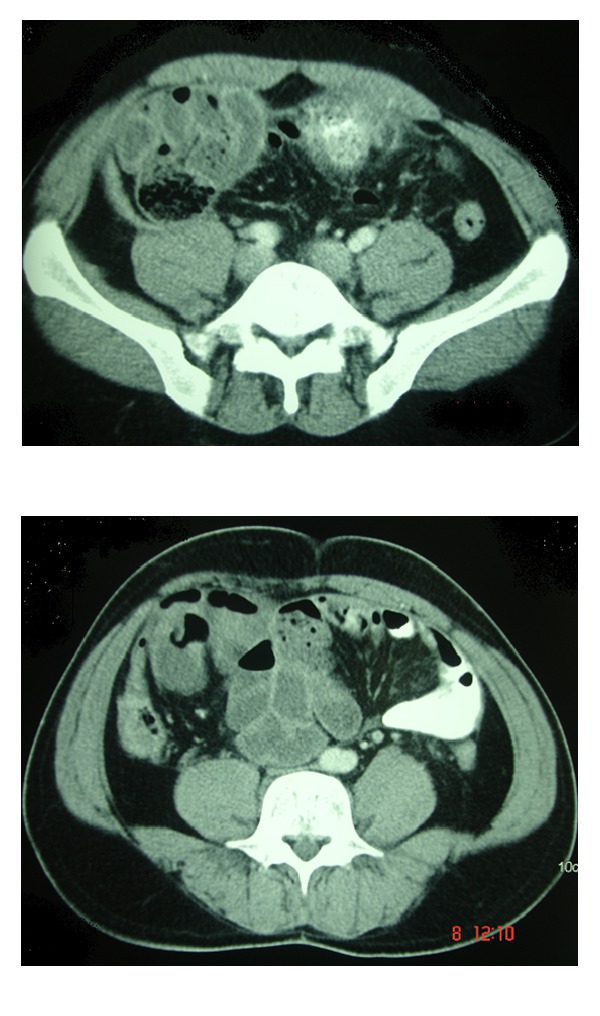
CT showing membrane enveloping loops of small bowel.
